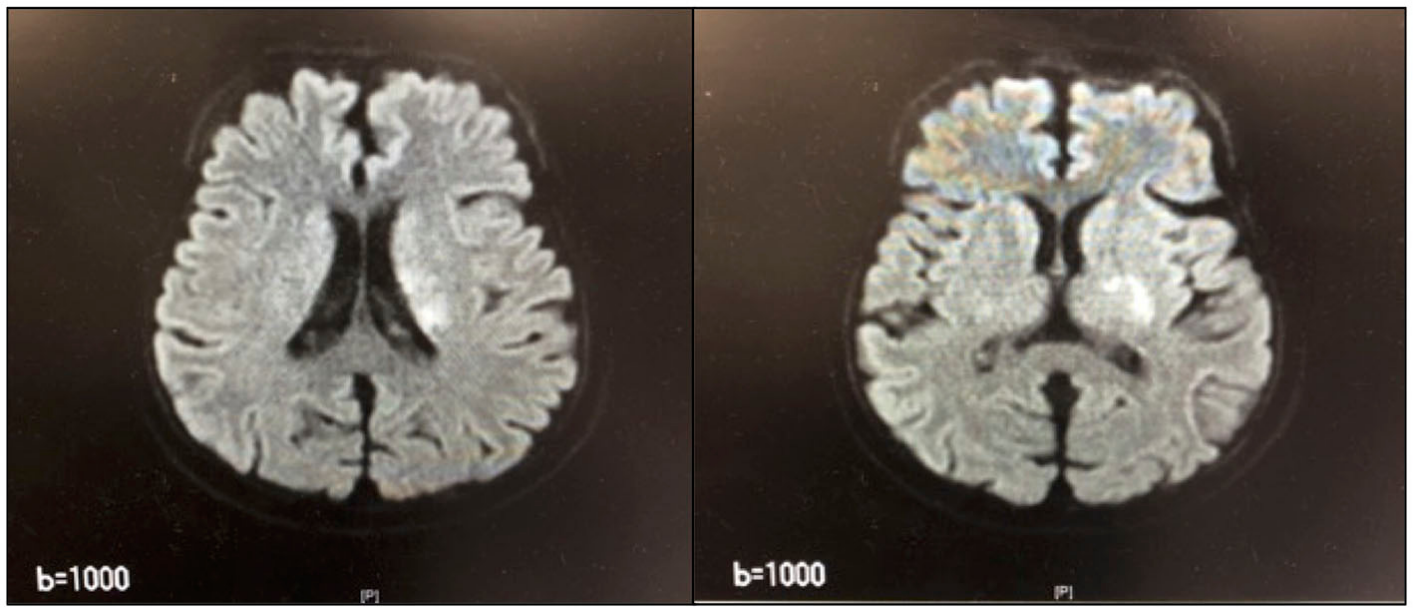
Magnetic Resonance Imaging of the head. High-signal area from the left parabasal corona to the inner retrosternal leg.
